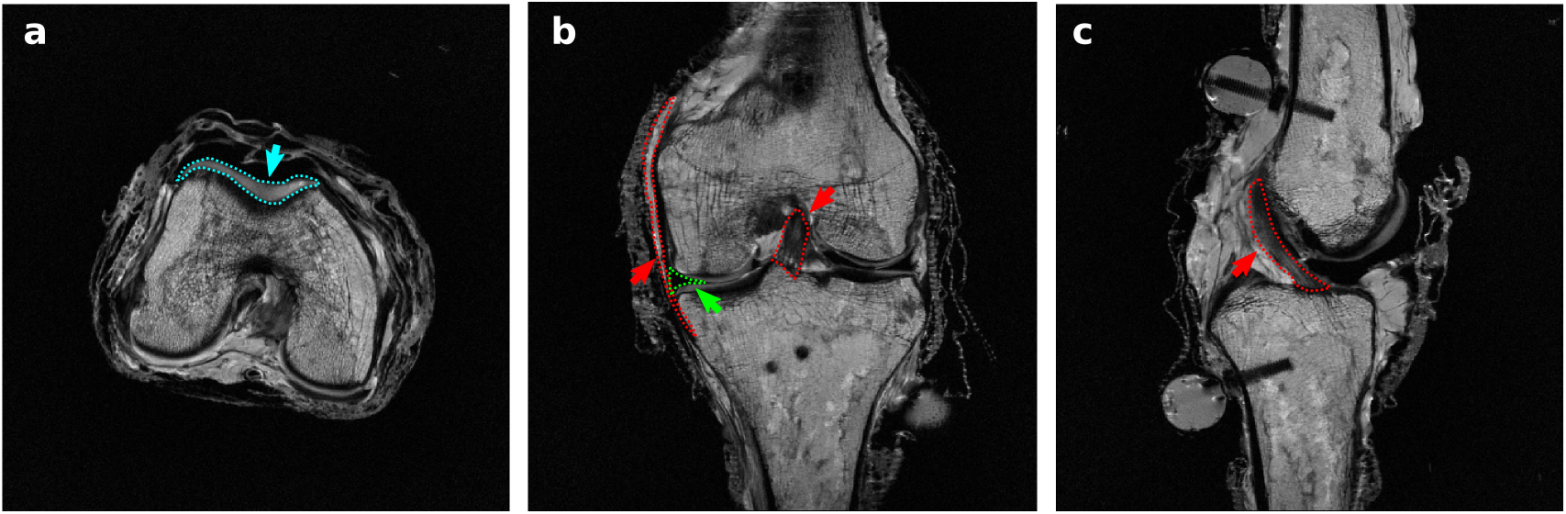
Magnetic resonance images of the tibiofemoral joint. a) Axial section; cartilage is highlighted. b) Coronal section; anterior cruciate ligament, medial collateral ligament, and medial meniscus are highlighted. c) Sagittal section; anterior cruciate ligament is highlighted.
